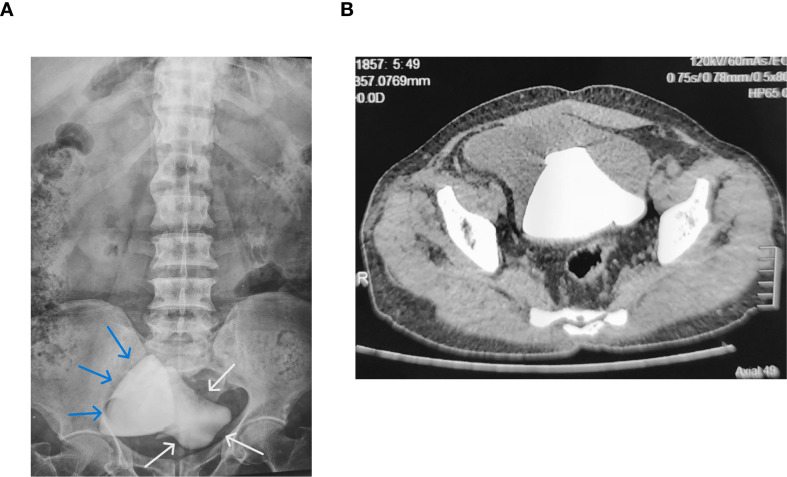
Preoperative imaging of giant neobladder stones. (A) Plain abdominal radiograph (KUB view): Two large radio-opaque calcific densities projecting over the neobladder region, consistent with two giant stones. (Blue and White Arrow) (B) Axial CT scan: Two high-density stones (1,300 Hounsfield Units) measuring 10×8×6 cm and 9×7×8 cm occupying most of the neobladder capacity.
